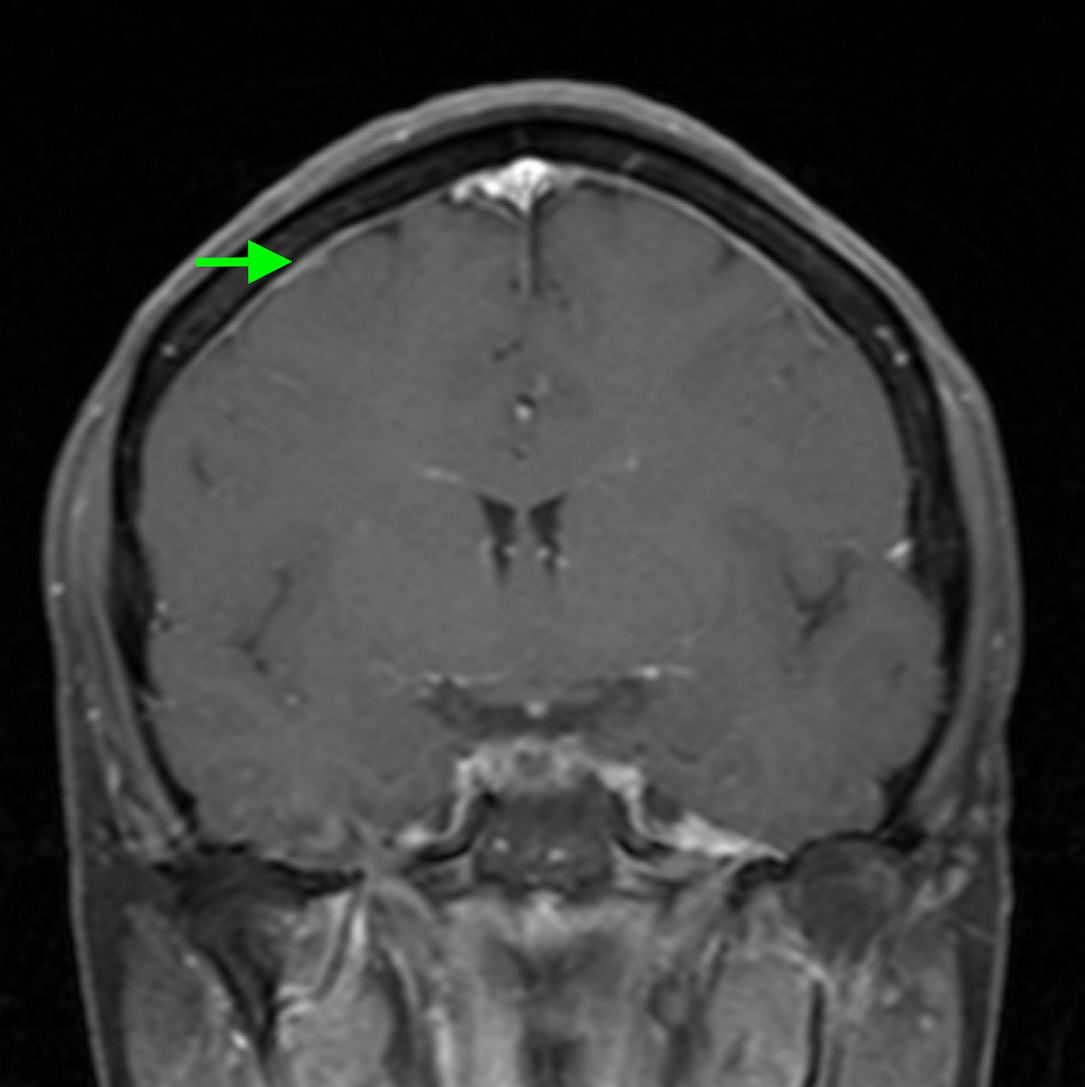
T1 coronal post-contrast MRI revealing enhancement of the convexity dura.
